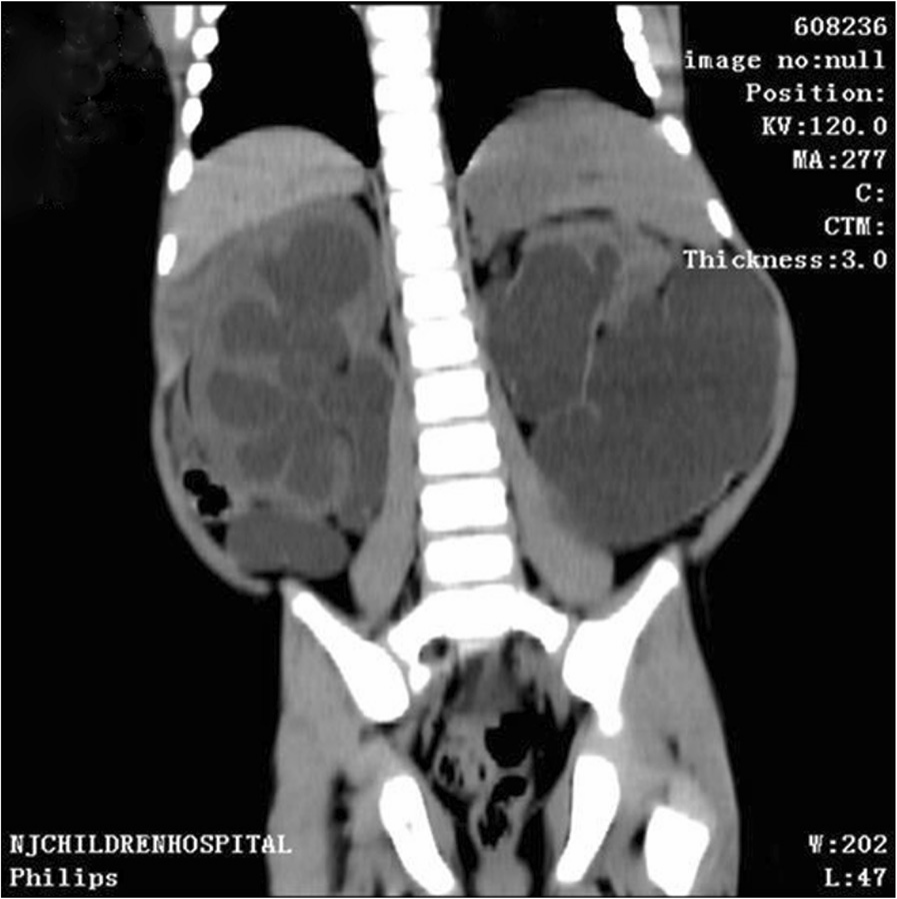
Abdominal computed tomography shows a giant ureter on the left side and right hydronephrosis with the whole right ureter dilatation
